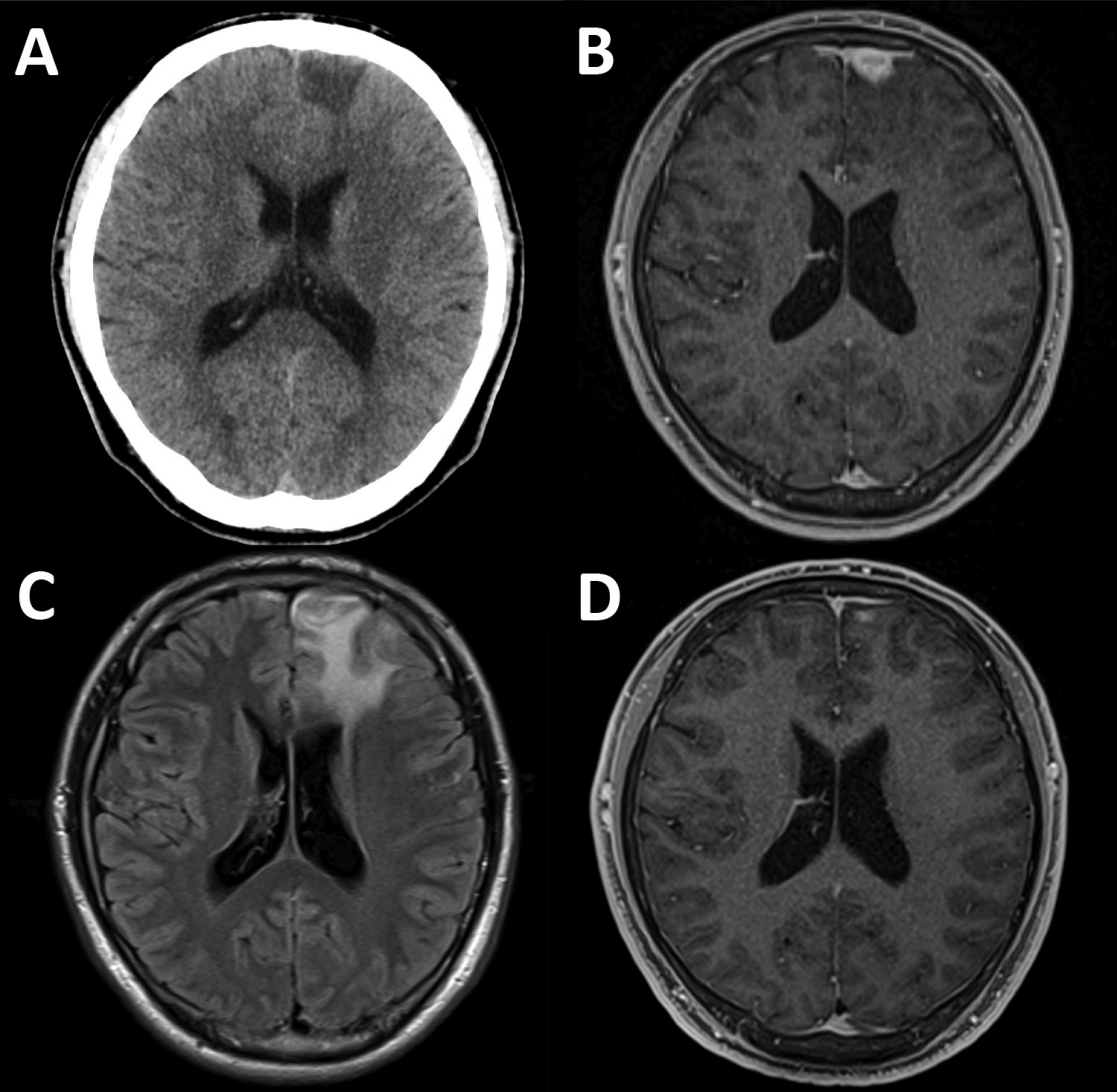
Diagnostic imaging results for a brain mass in a 21-year-old HIV-positive man with cerebral syphilitic gumma in Tokyo, Japan, for whom serum samples obtained as recently as 5 months earlier showed negative results for syphilis. A) Noncontrast, cranial computed tomography showing a hypodense lesion in the left frontal lobe. B) Gadolinium-enhanced, axial, T1-weighted magnetic resonance imaging (MRI) showing an enhanced lesion (mass) (14 × 14 × 12 mm) adjacent to the enhanced dura in the left frontal lobe. C) Axial, fluid-attenuated inversion recovery MRI showing extensive left frontal edema. D) Two months after treatment for syphilis, gadolinium-enhanced, axial T1-weighted MRI showing substantial resolution of the lesion.
